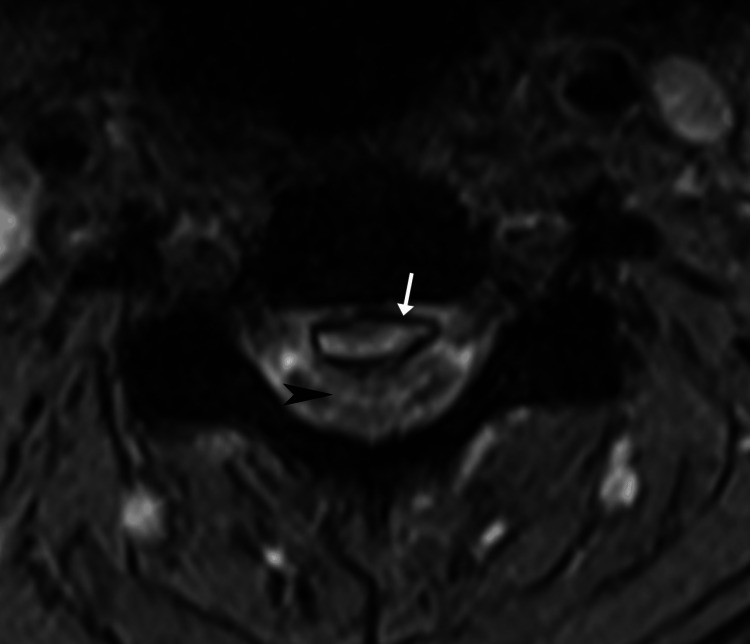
Cervical magnetic resonance imaging, T2-weighted axial at the level of C3/4 The epidural layer is bordered by a T2-hypointense rim (arrow), suggestive of hemosiderin deposition. The spinal cord is markedly impressed, and cord edema is present (black arrowhead).
